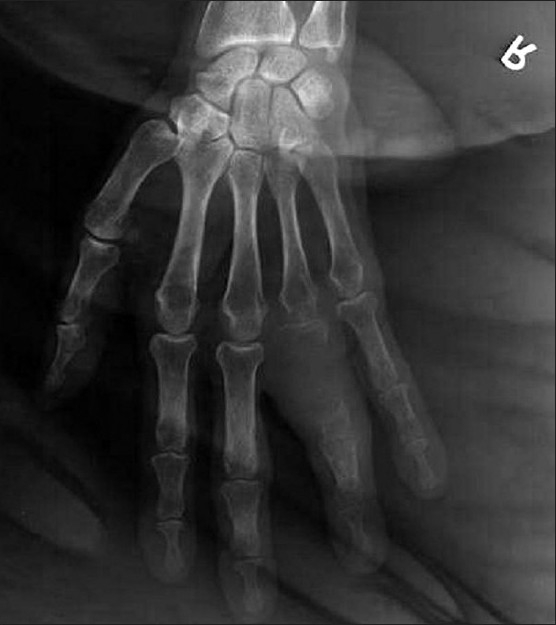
X-ray showing destruction of the first phalanx of the ring finger
